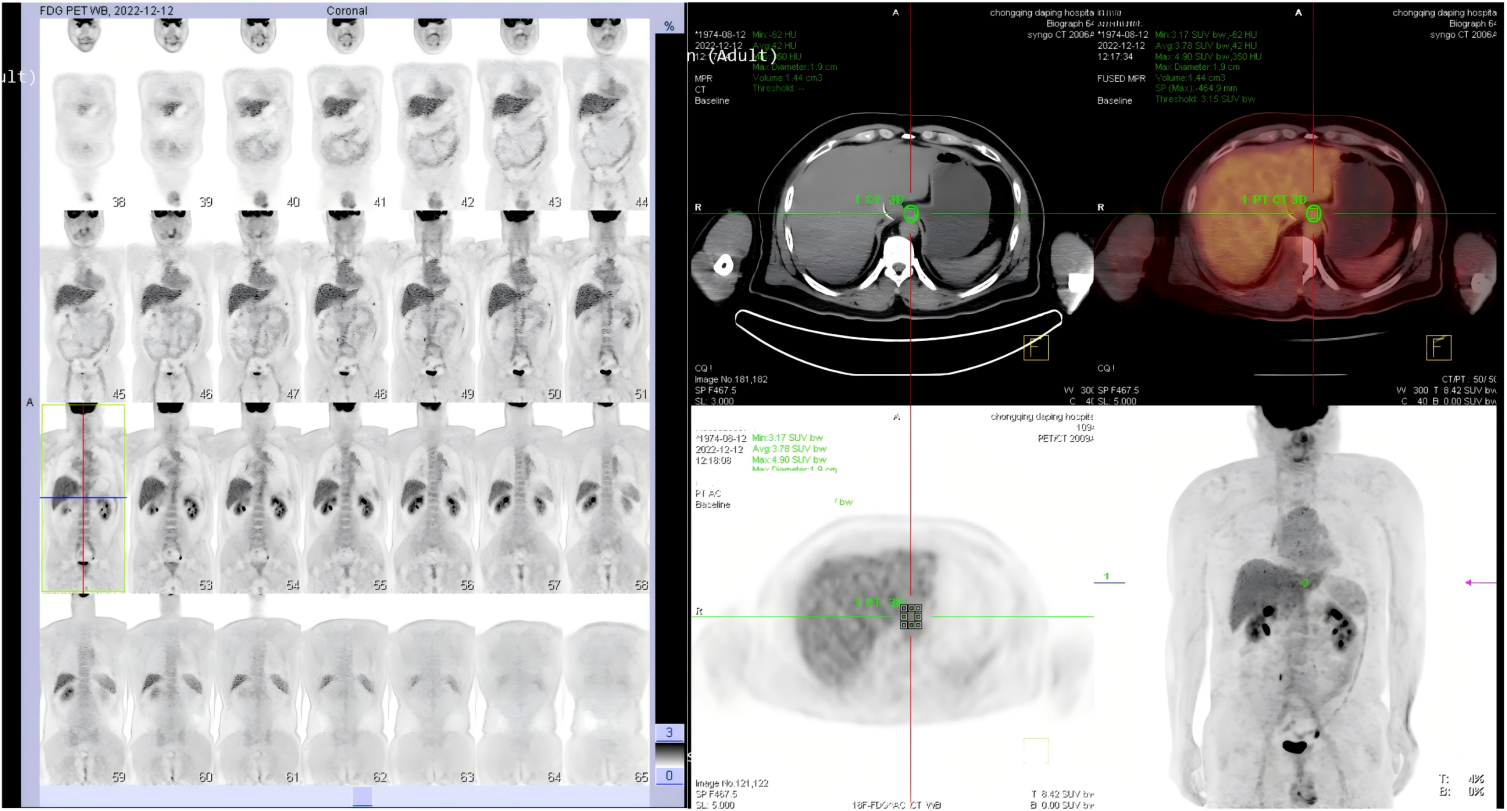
Treatment response assessment. (A, B) Fused PET-CT images at the level of the esophagogastric junction before (A) and after (B) neoadjuvant therapy, showing significant metabolic regression of the primary tumor (SUVmax reduced) and complete resolution of previously noted liver metastases, indicating a complete clinical response (CR).
